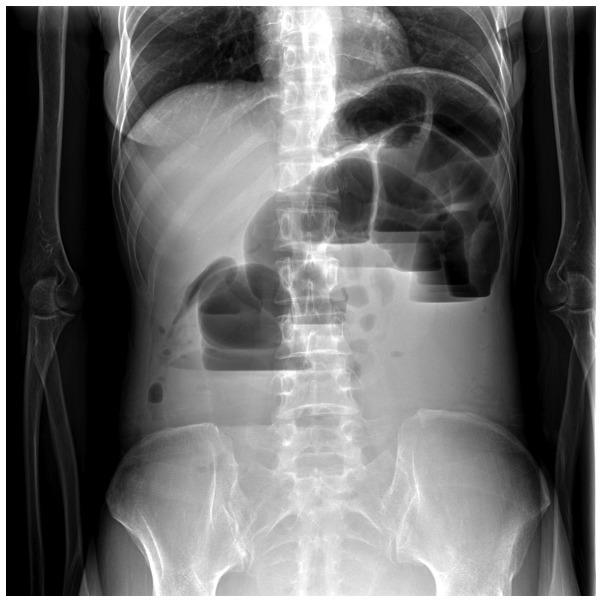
Abdominal X-ray revealing multiple liquid-gas surfaces.
